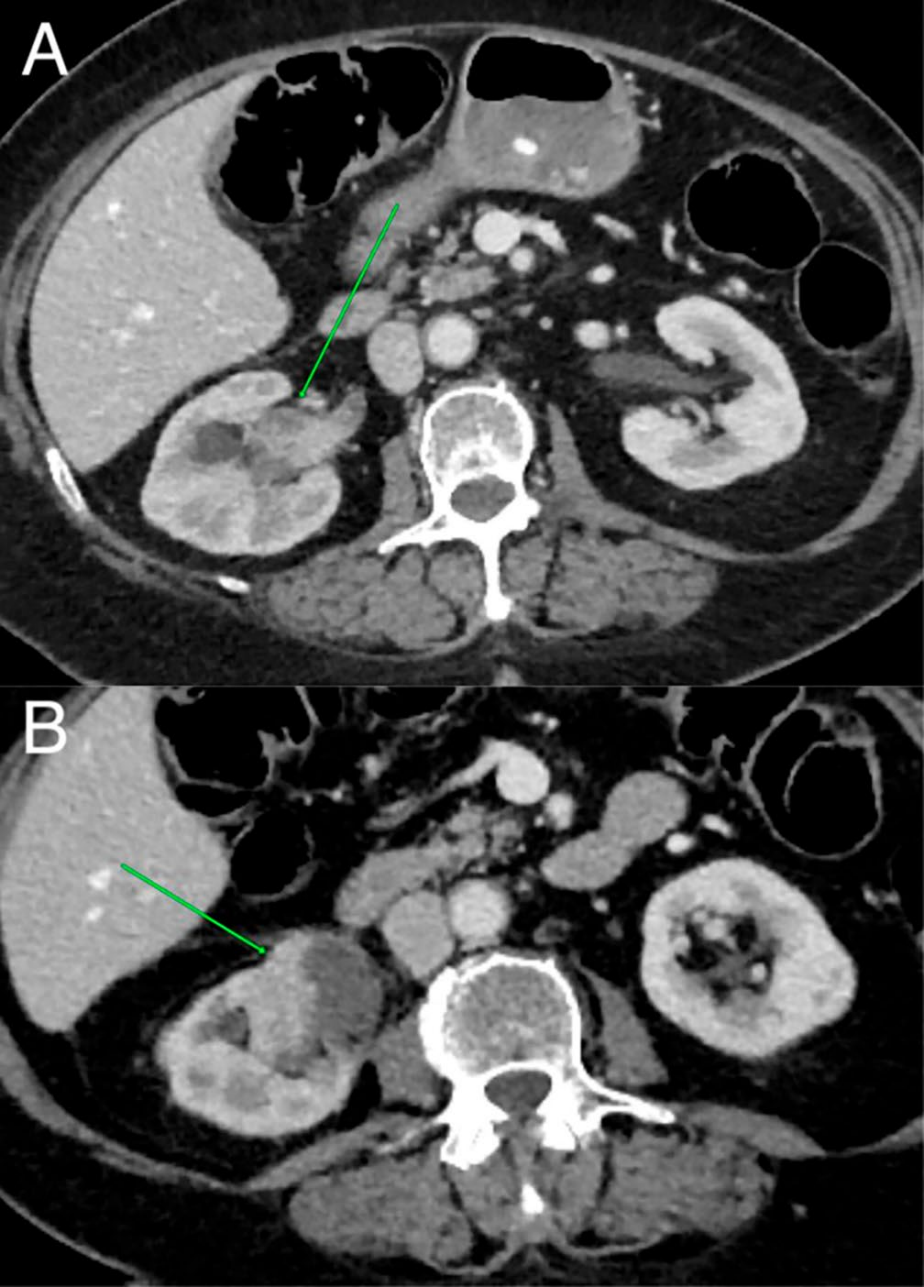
Abdominal computed tomography 2 years after cryoablation. A viable tumor is depicted expanding into the renal pelvis (A) as well as the periphery of the previously ablated tumor (B).
